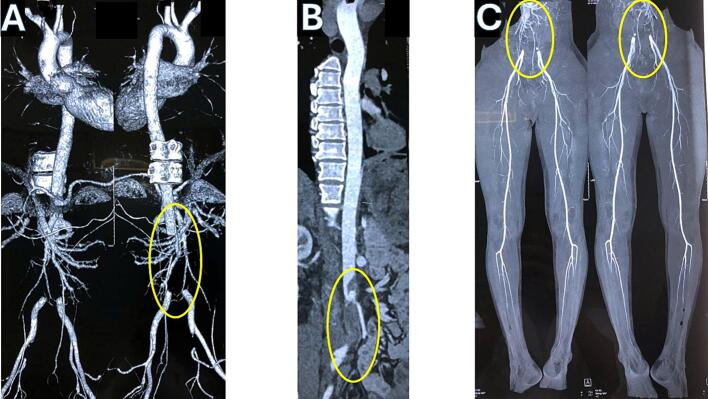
Computed tomography (CT) angiography of the aorta–iliac and lower limb arterial systems. (A-B) Contrast-enhanced CT with 3D reconstruction demonstrates complete occlusion of the infrarenal abdominal aorta and bilateral common iliac arteries (yellow ellipses). (C) In contrast, the lower limb arterial system shows no evidence of obstruction, indicating preserved distal perfusion via collateral circulation. (For interpretation of the references to colour in this figure legend, the reader is referred to the web version of this article.)
